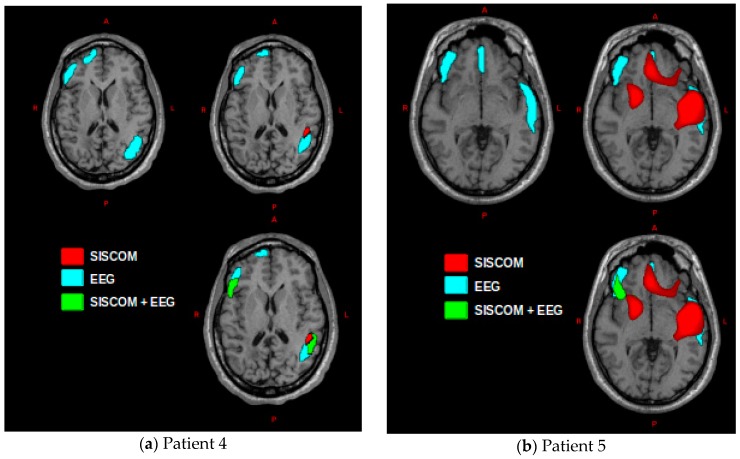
EZ estimated by the proposed methodology and co-registered to T1-weighted MRI: SISCOM (in red), ictal ESI (blue) and ictal ESI using SISCOM as prior (green); (a) for patient 4; and (b) for patient 5.
